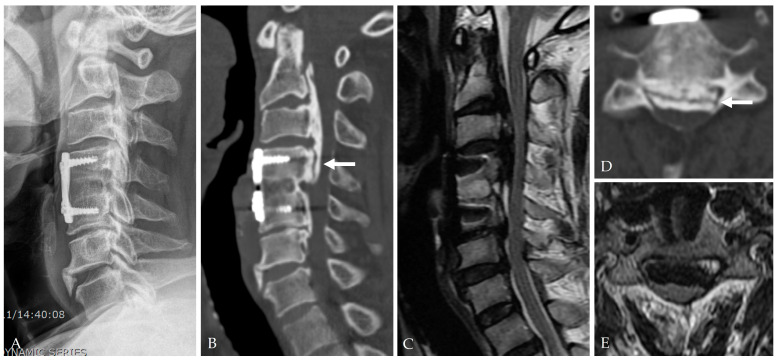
A 66-year-old male patient complained of cervical myelopathy. He underwent C4–5 ACDF 22 years ago. Latreal X-ray (A), CT (B,D), and MRI (C,E) showed C2–7 OPLL compressing the spinal cord and ‘double-layer’ sign (white arrow).
